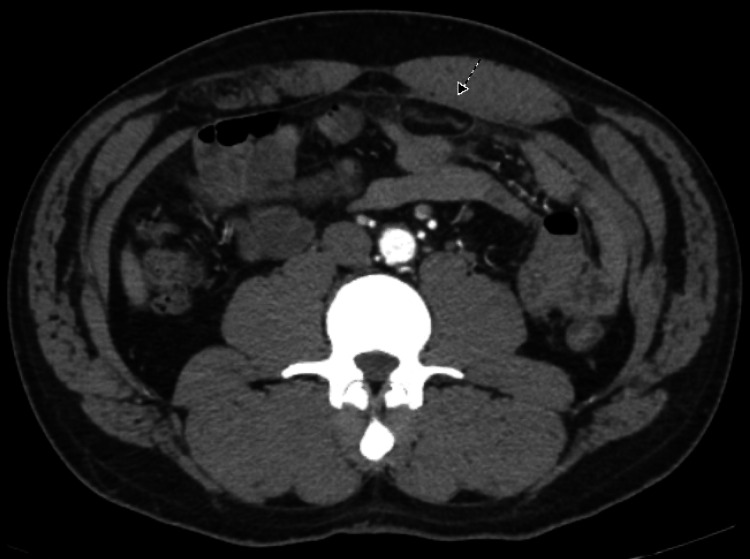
Computed tomography of the abdomen The arrow points to the 3.8 cm sharply circumscribed fat attenuation structure in axial view. It is surrounded by mild inflammation and is located in the anterior left mid-abdomen along the transverse colon.
